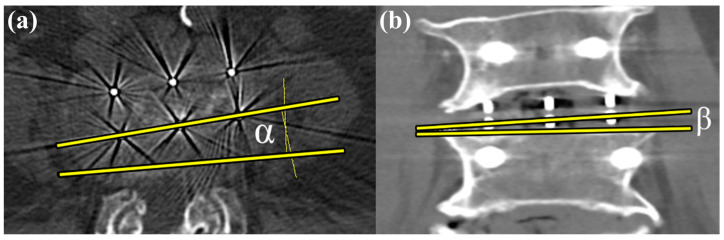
Measurement of cage insertion angles. (a) In the axial plane, the angle (α) formed between the long axis of the disc space and the long axis of the cage, measured using radiographic markers, is defined as the axial plane insertion angle. Positive angles are assigned to cages inserted from the left anterior to the right posterior position. (b) In the coronal plane, the angle (β) formed between the line parallel to the L5 upper endplate and the long axis of the cage, measured using radiographic markers, is defined as the coronal plane insertion angle. Negative angles are assigned to cages inserted from the left cranial to the right caudal direction.
